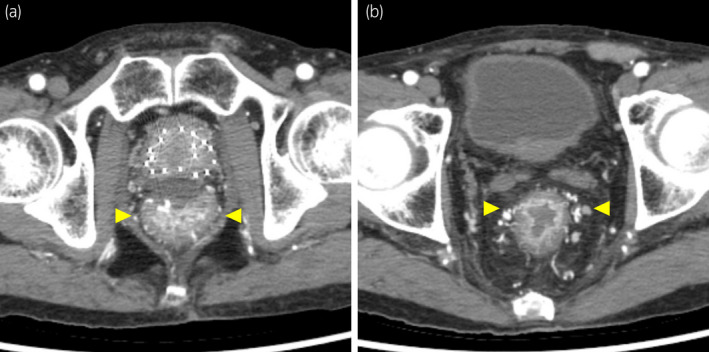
Contrast‐enhanced computed tomography at rehospitalization. (a) The spacer was still properly present. (b) Proctitis was suspected because of increased blood flow (yellow arrowheads) of the rectal mucosa. [Colour figure can be viewed at wileyonlinelibrary.com]
